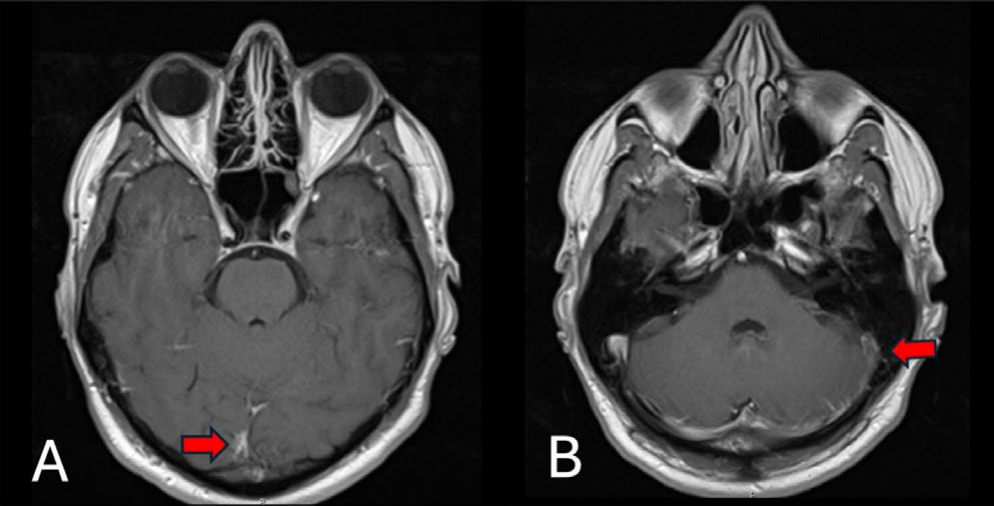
(A) T1‐post contrast axial MRI showed very thin central filling defect (the red arrow) along the superior sagittal sinus and (B) T1‐post contrast axial MRI showed filling defect (the red arrow) along the left transverse and sigmoid sinuses as well as the internal jugular vein denoting acute sinus thrombosis.
